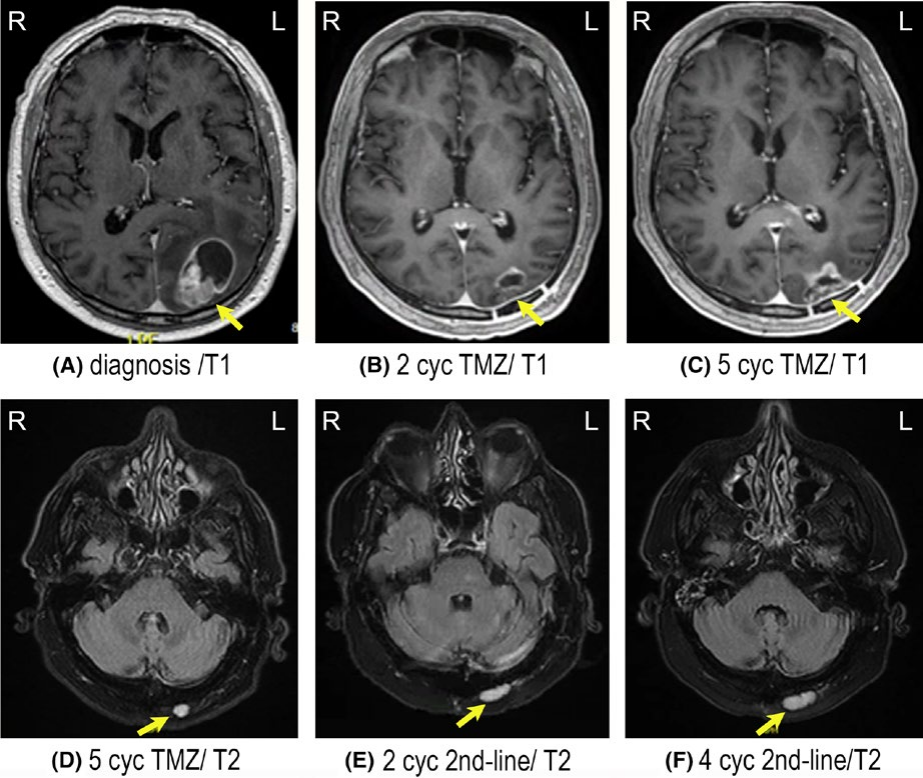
Tumor imaging of Case 2. A, Preoperative T1‐weighted MRI showing a cystic, multi‐lobular, contrast‐enhanced 2.5 × 4.7 cm large lesion in the left parietal lobe. B, Stable disease (SD) after two series of adjuvant temozolomide (TMZ) on T1‐weighted MRI. C and D, Progressive disease (PD) after five cycles of adjuvant TMZ with a new lesion in the neck on T1 and T2‐FLAIR MRI. E, T2‐FLAIR MRI after two cycles of bevacizumab (BEV)/CPT11, SD at the primary tumor site but PD in the tumor in the neck. F, T2‐FLAIR MRI after four cycles of BEV/CPT‐11 finding SD at the primary tumor site but PD of the tumor in the neck. Body orientation is indicated by R (right) and L (left)
